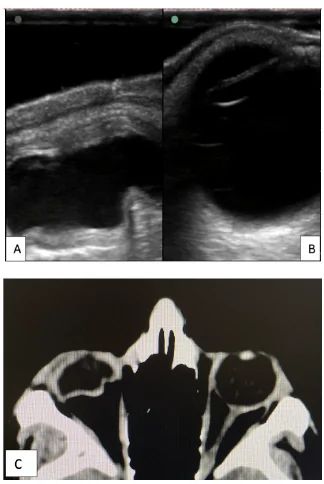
Case 2. Ocular ultrasound modified water bath technique performed: A) right globe injury with loss of globe volume and decrease in size; B) normal left eye; and C) computed tomography findings suggestive of right globe injury.
